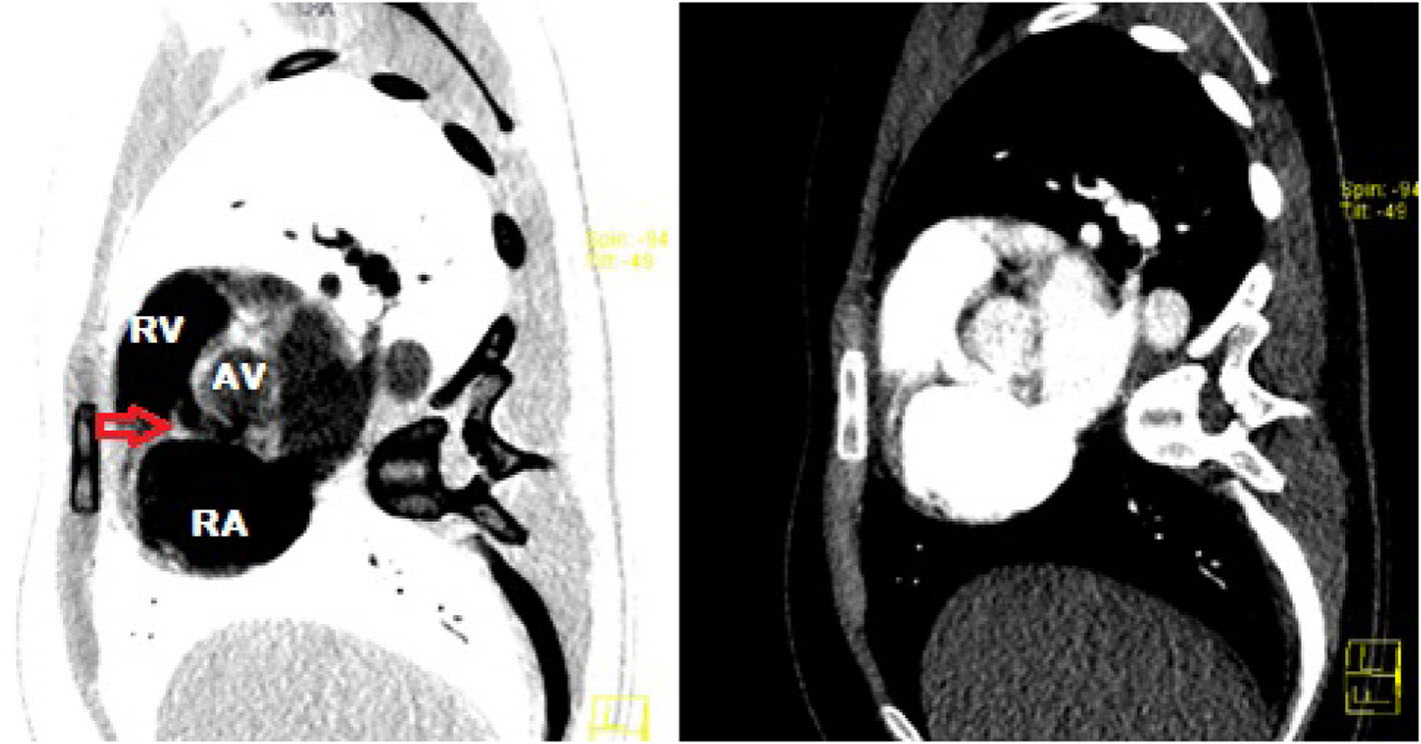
Reconstructed computed tomography pulmonary angiography sagittal view image shows intravenous contrast in the communication between the aortic root and probable right ventricle (red arrow). RV, right ventricle; RA, right atrium; AV, aortic valve
